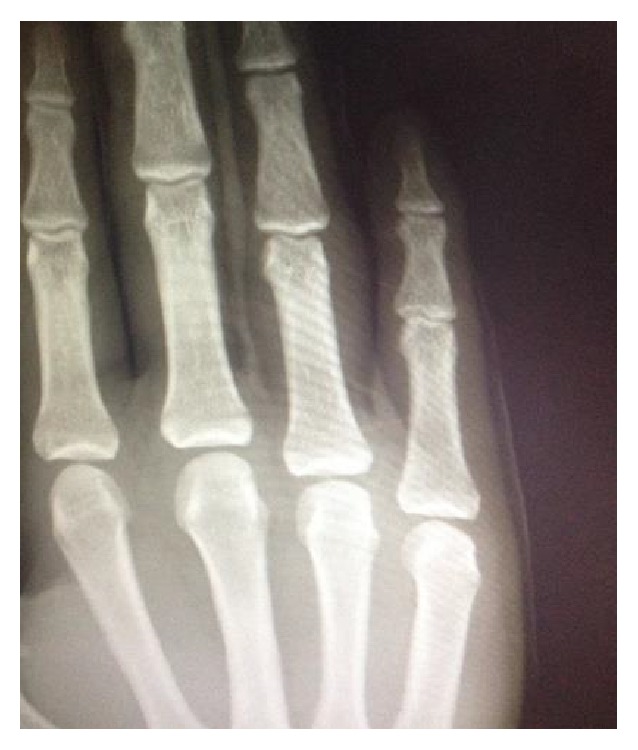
X-ray showing reduced finger.
